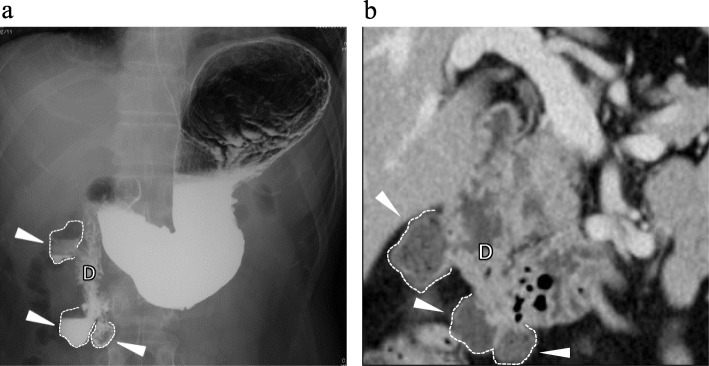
An upper gastrointestinal series and a contrast-enhanced CT of case 2 before gastrectomy. Multiple non-ampullary duodenal diverticula (white arrowhead) were detected at the second and third parts of the duodenum (D) (a, b)
